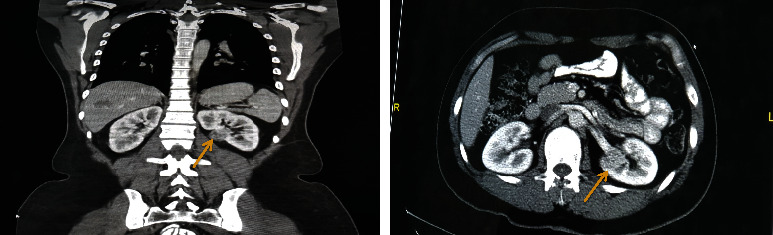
Axial and coronal view of abdominal CT scan showed a well-defined hypodense focal lesion originally from left kidney near hilum region with clue sign. The renal lesion showed inhomogeneous enhancement in the arterial phase with partial wash out in delayed phase, and it measured 2.6 cm × 2.6 cm.
